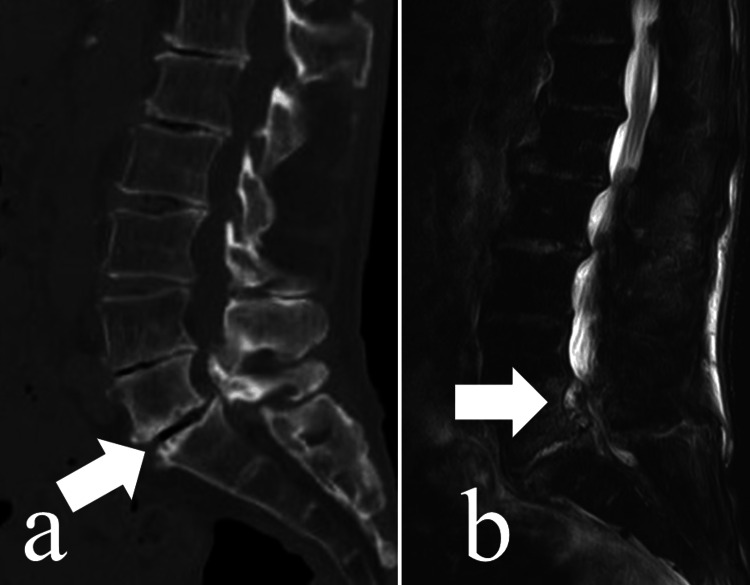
Preoperative CT and MRI images a. CT before surgery. Lytic lesions were detected in the L4/5 intervertebral disc (white arrow). b. MRI before surgery. An abscess was detected at L4/5 (white arrow).
